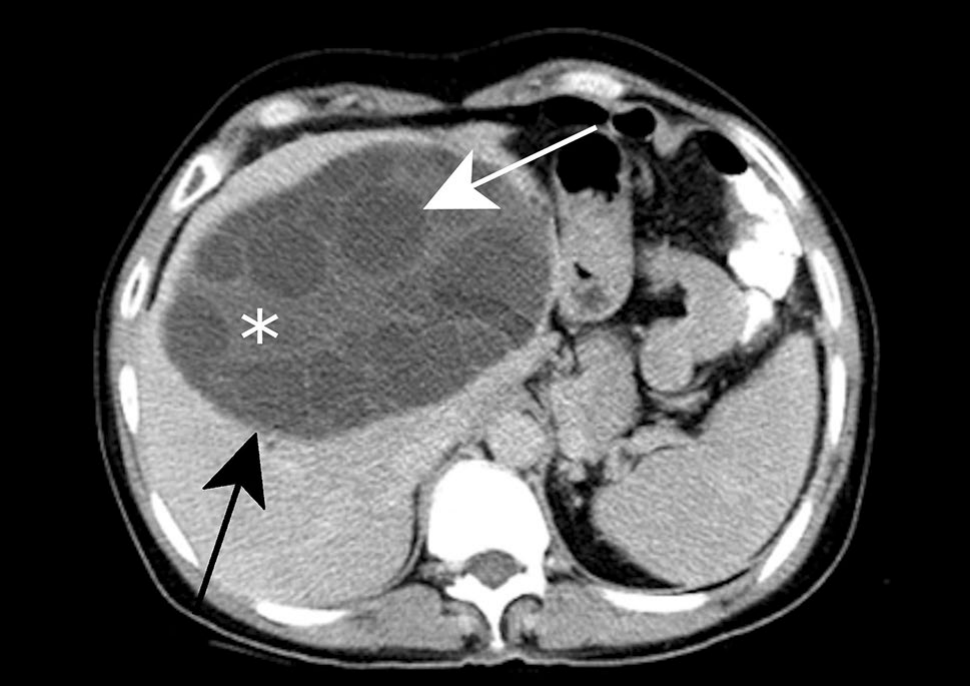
Cystic echinococcosis type CE3b in a 54-year-old male patient with right upper quadrant pain. Axial contrast-enhanced CT reveals a large hepatic cyst in right hepatic lobe (black arrow) with solid matrix in the center (asterisk) and peripherally located daughter cysts (white arrow)
